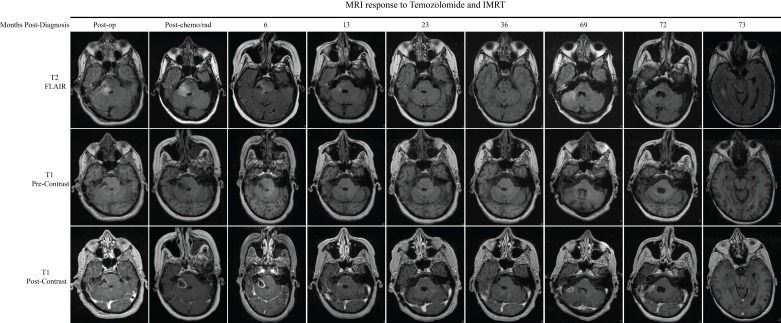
MRI response of tumor growth to temozolomide and radiation therapy Serial MRI scans of the brain showing response of the lesion to chemotherapy and radiation. Recurrence in the right cerebellum is noted at 69 months. Minimal ependymal enhancement can be seen in the right temporal horn of the ventricle at 73 months.
